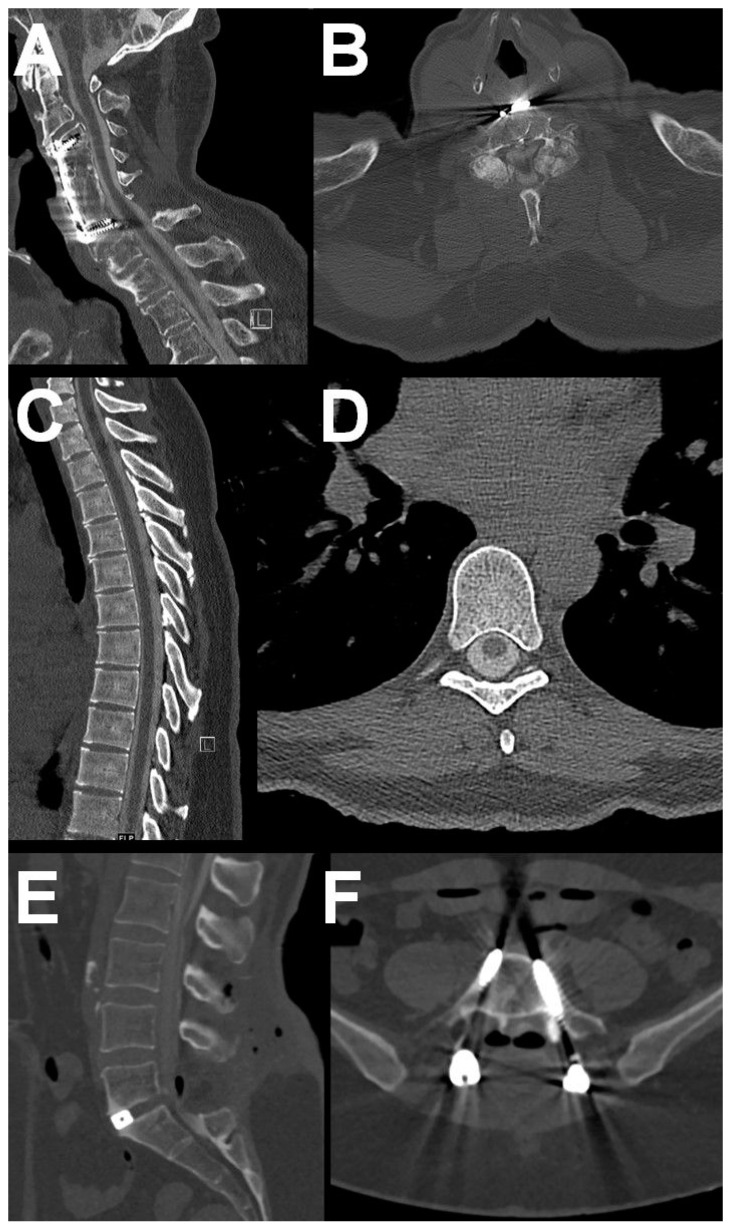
Image examples of CT myelography (CTM) of specific parts of the spine on the different CT scanners. (A) + (B): CTM on the SOMATOM Definition Flash of the cervical spine of a 79-year-old patient after ventral fusion C3–6 with impaired fine motor function of both hands with high-grade stenosis of neuroforamina C3 and C4 in the presence of marked hypertrophic spondylo-arthrosis. (C) + (D): Inconspicuous CTM on the SOMATOM Definition AS+ of the thoracic spine in a 41-year-old female patient with suspected thoracic CSF cyst. (E) + (F): CTM in dual-source technique on the SOMATOM Force of the lumbar spine of a 44-year-old female patient with a CSF leak at the level of L5 on the right after dorsal spondylosis and insertion of a disc cage. All scanners: Siemens Healthineers, Forchheim, Germany.
